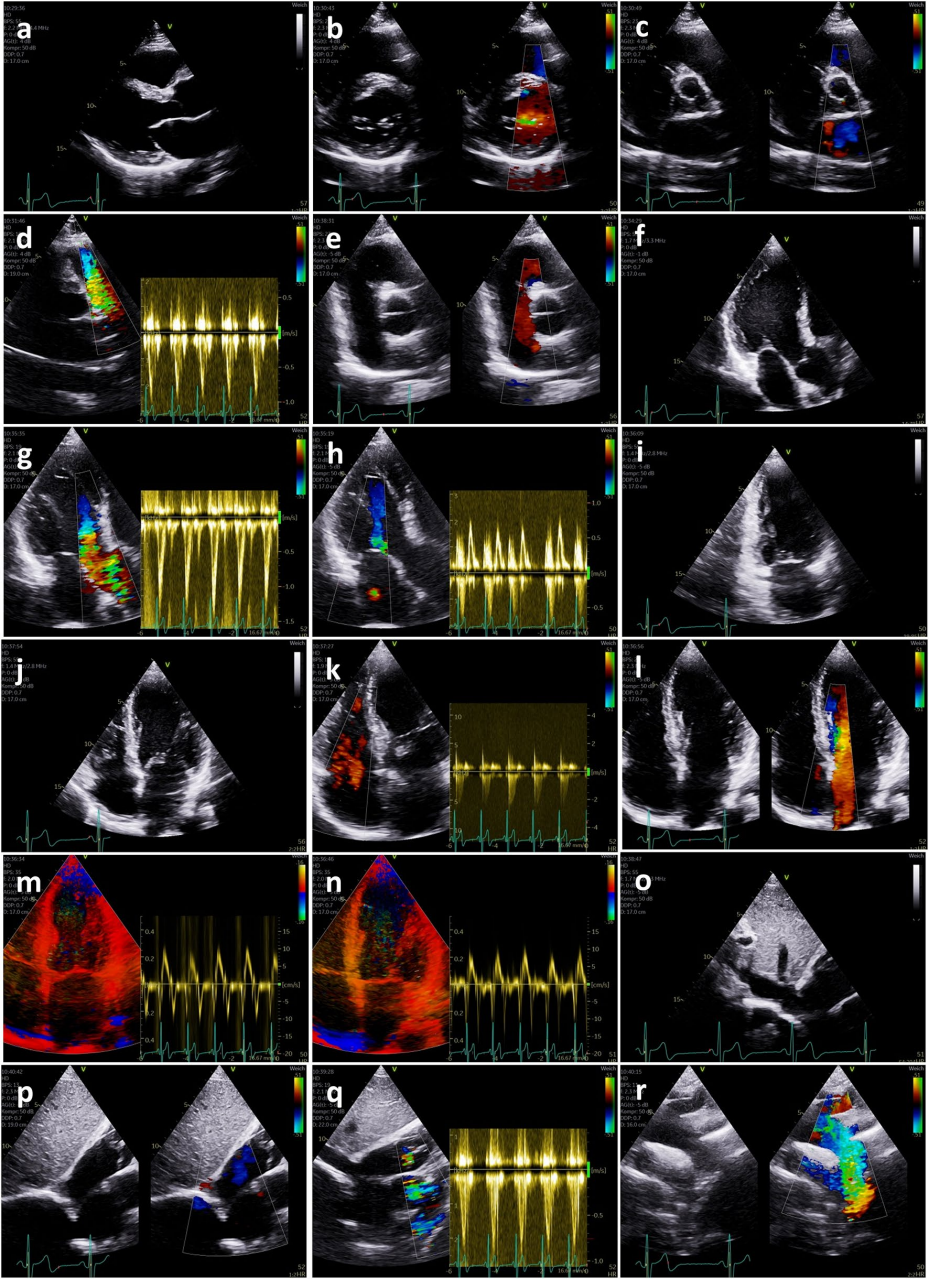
Image acquisition transthoracic echocardiography protocol in post-stroke patients. Parasternal long axis view for measurements of left ventricular (LV) diameters, left ventricular outflow tract (LVOT) diameter, LV-wall thickness, dimensions of the aortic arch (a); parasternal short axis view at the level of the mitral valve (MV) for assessment of MV pathologies, detection or exclusion of MV stenosis or MV regurgitation (b); parasternal short axis view at the level of the aortic valve (AV) for assessment of AV pathologies, detection or exclusion of AV stenosis or AV regurgitation (c); parasternal short axis view at the level of the pulmonary valve (PV) and pulsed wave (pw) Doppler spectrum of the right ventricular (RV) outflow tract (RVOT) flow to assess RV stroke volume for pulmonary stroke volume (Qp)/ systemic stroke volume (Qs)—calculation (d); parasternal short axis view of the interatrial septum to detect or exclude atrial communication defects (e); apical long axis view for assessment of LV function using deformation imaging (f); color-coded apical long axis view for assessment of AV function including pw Doppler spectrum of the LVOT flow to assess LV stroke volume for Qp/Qs-calculation (g), if AV is pathological a continuous wave (cw) Doppler spectrum has to be added; color-coded apical long axis view for assessment of MV function including pw Doppler spectrum of the transmitral flow (h), if MV is pathological a cw Doppler spectrum has to be added; apical 2-chamber view for assessment of LV function using deformation imaging (i); apical 4-chamber view for assessment of LV function using deformation imaging (j); color-coded apical 4-chamber view for assessment of tricuspid valve function including cw Doppler spectrum to assess systolic pulmonary artery pressure (sPAP) (k); color-coded apical 4-chamber view of the interatrial septum to detect or exclude atrial communication defects (l); color-coded tissue Doppler apical 4-chamber view including tissue pw Doppler spectrum of the basal septal myocardial velocities (m); color-coded tissue Doppler apical 4-chamber view including tissue pw Doppler spectrum of the lateral septal myocardial velocities (n); subcostal view of the inferior caval vein to document systemic volume state (o); subcostal short axis view of the interatrial septum to detect or exclude atrial communication defects (p); subcostal short axis view at the level of the pulmonary valve and pw Doppler spectrum of the RVOT flow to assess RV stroke volume for Qp/Qs-calculation, if parasternal view is not possible (q); suprasternal view of the aortic arch to detect or exclude aortic dissection and other aortic pathologies (r). *AV* aortic valve, *cw* continuous wave, *LV* left ventricular, *LVOT* left ventricular outflow tract, *MV* mitral valve, *pw* pulsed wave, *Qs* systemic stroke volume, *Qp* pulmonary stroke volume, *RV* right ventricular, *RVOT* right ventricular outflow tract
